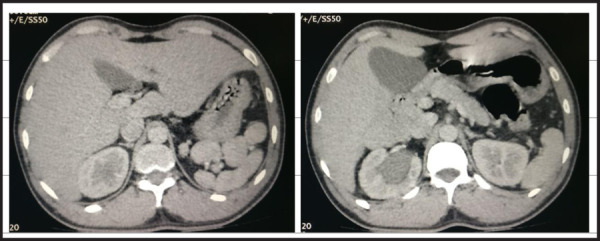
Axial CT scans show multiple accessory spleens and short pancreas. Hydronephrosis in right kidney.
